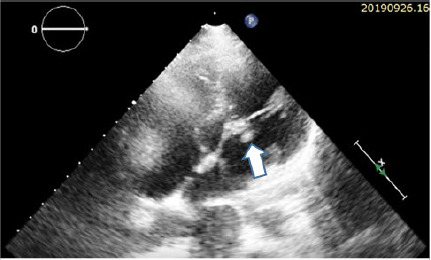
Echocardiography (subxiphoid view) showing vegetation in the anterior mitral leaflet.
